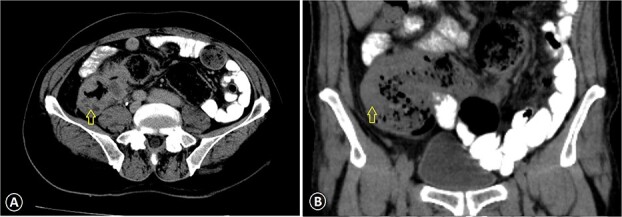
Ascending colon cancer. The CT image with IV contrast revealed a markedly thickened colonic wall (arrow) with pericolonic inflammation: (A) axial view CT scan and (B) coronal view CT scan.
